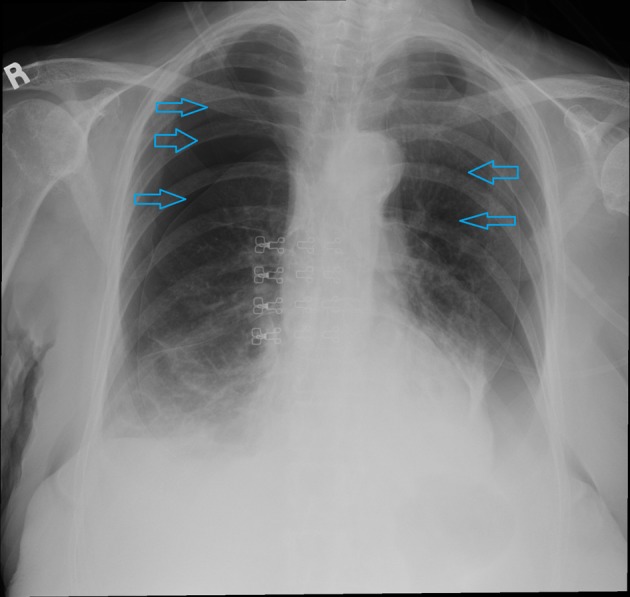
Chest X-ray demonstrating bilateral pneumothoraces.
